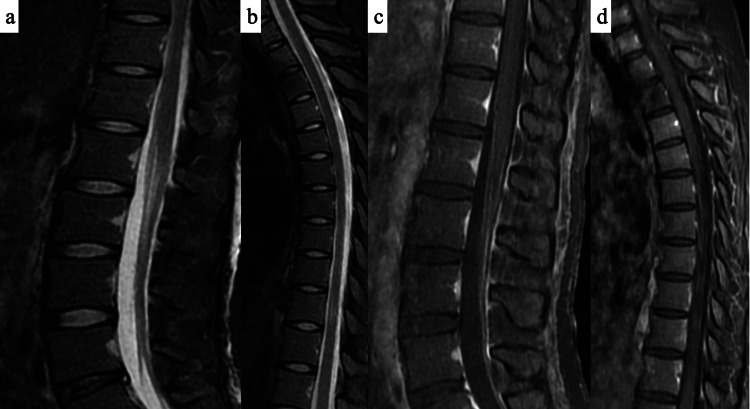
Magnetic resonance imaging findings at three days postoperatively A T2-weighted magnetic resonance imaging (MRI) scan showed no significant abnormal signals in the vertebrae or intervertebral discs (a: lumbar, b: thoracic). A contrast-enhanced MRI scan observed no abnormal enhancement or contrast agent leakage into the epidural space (c: lumbar, d: thoracic).
